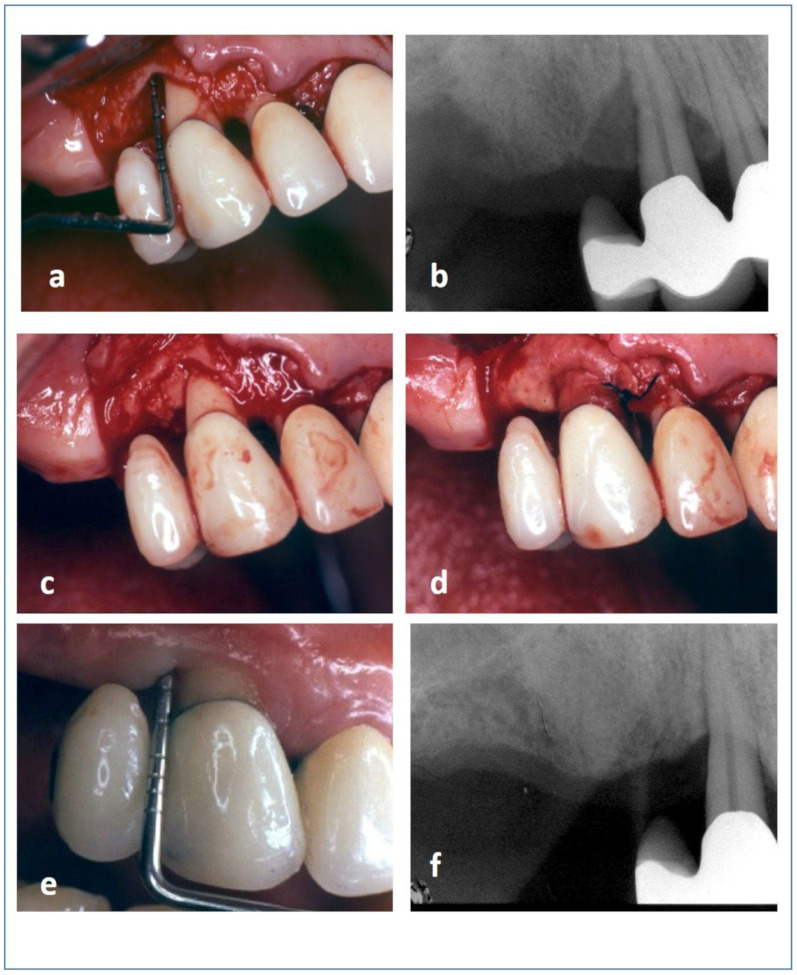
(a) The intrabony defect; (b) X-ray appearance of the defect; the intrabony defect was (c) filled with ABG and (d) covered with a CM; (e) clinical and (f) radiographic appearance of the treated site 1 year after surgery.
